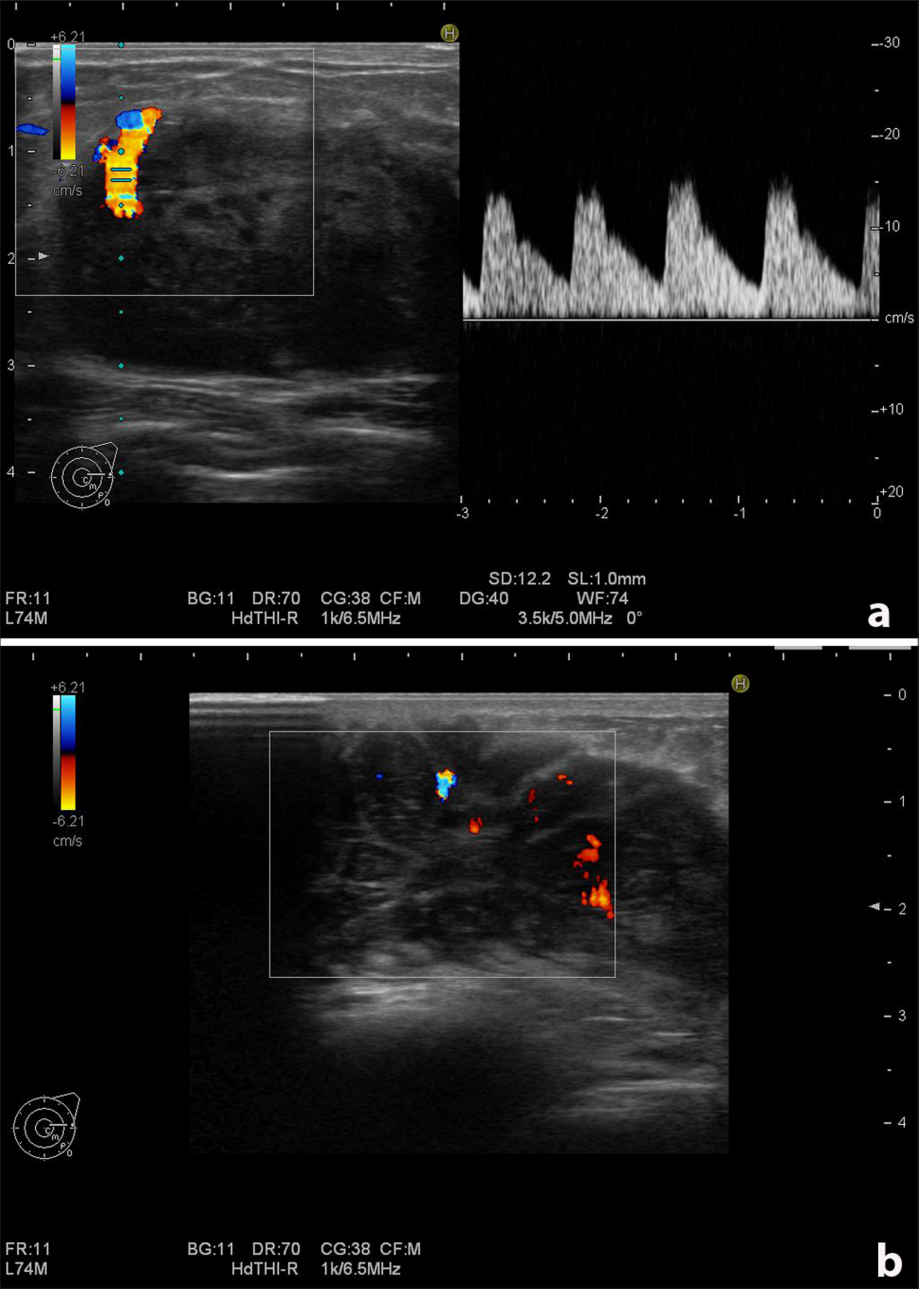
Doppler examination of the largest mass on the right breast. (a) Increased vascularity at the contours of the mass. (b) Increased vascularity in the internal part of the mass.
